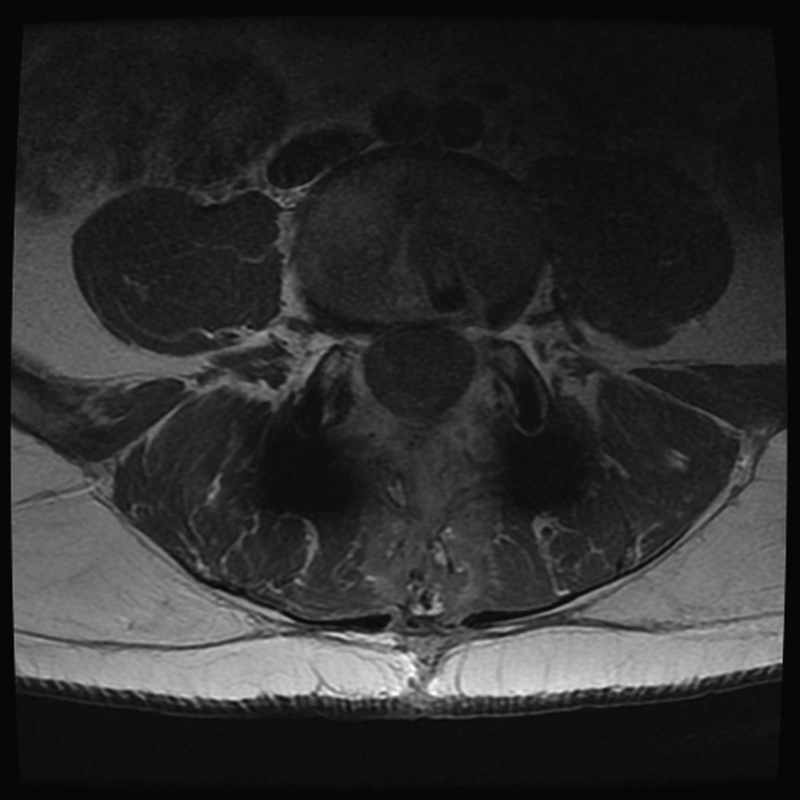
Axial magnetic resonance imaging with contrast 1 year after tumor resection demonstrating no evidence of tumor recurrence. The patient had undergone L4–L5 fusion elsewhere prior to tumor resection.
